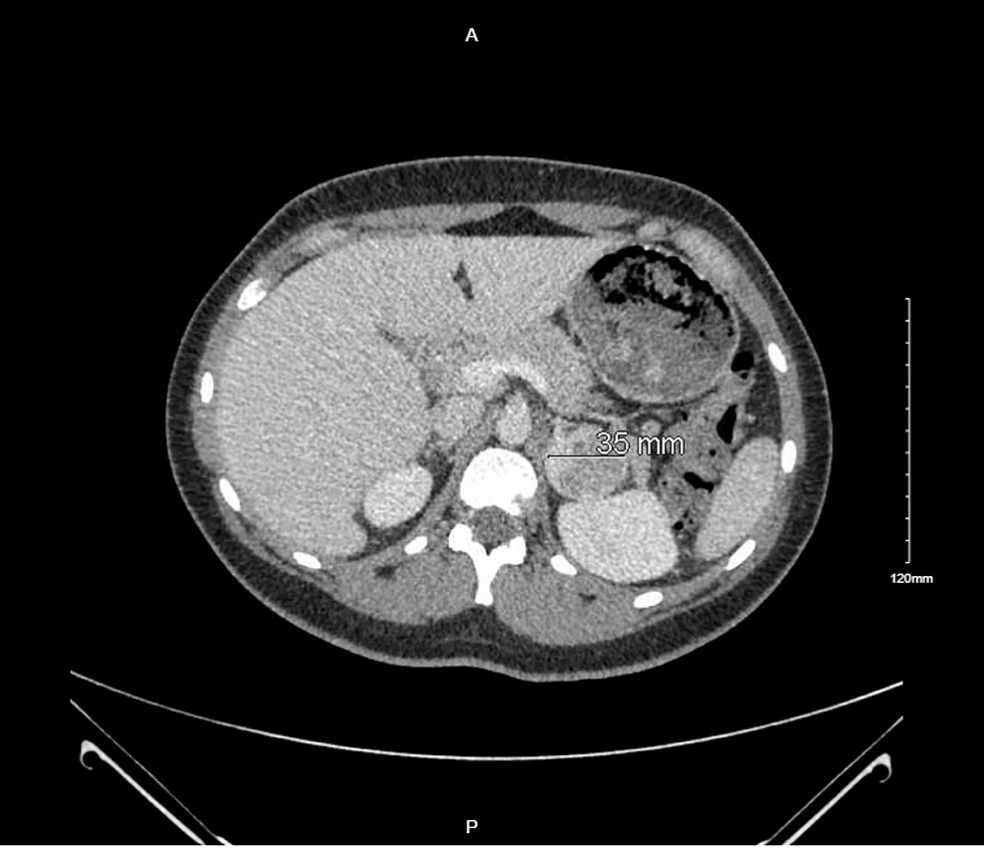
CT abdomen with and without contrast showing a left adrenal heterogeneously enhancing nodule measuring 35 x 68 x 35 mm
